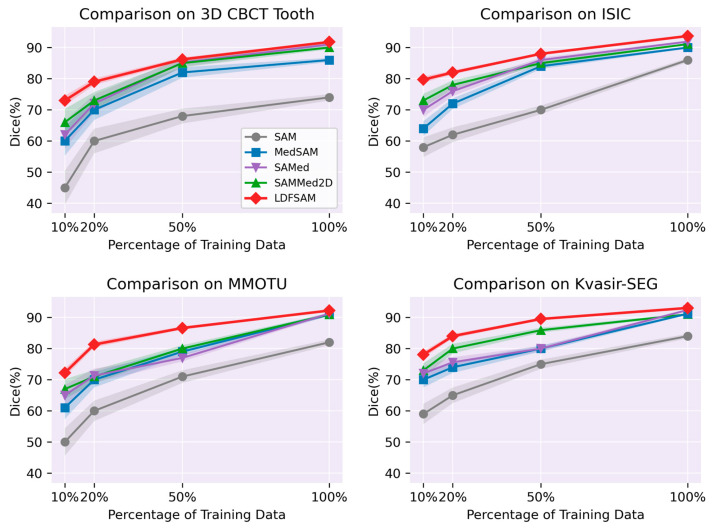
Comparison with SAM-based methods under different annotation budgets. Dice scores of SAM, MedSAM, SAMed, SAM-Med2D and LDFSAM on 3D CBCT Tooth, ISIC 2018, MMOTU, and Kvasir-SEG, using 10%, 20%, 50% and 100% of the training masks. Shaded bands represent 95% bootstrap confidence intervals over five fixed-seed runs.
